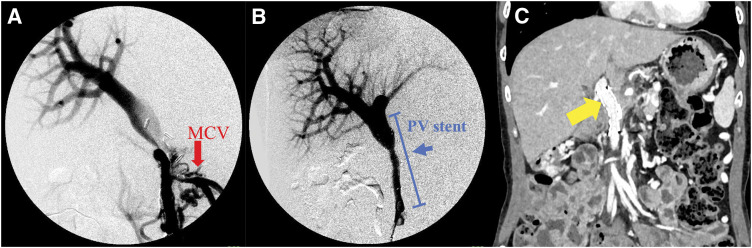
The patient underwent PV stenting via the ileocecal approach. (A) Portography before stenting. The left branch of the PV was not contrasted at all, and the MCV was contrasted. (B) Portography after stenting. The left branch of the PV was slightly contrasted, and the MCV was no longer contrasted. (C) Enhanced CT performed 2 years after PV stenting showed no stent migration or occlusion (arrow) and no recurrence of esophageal varices. MCV, middle colic vein; PV, portal vein
